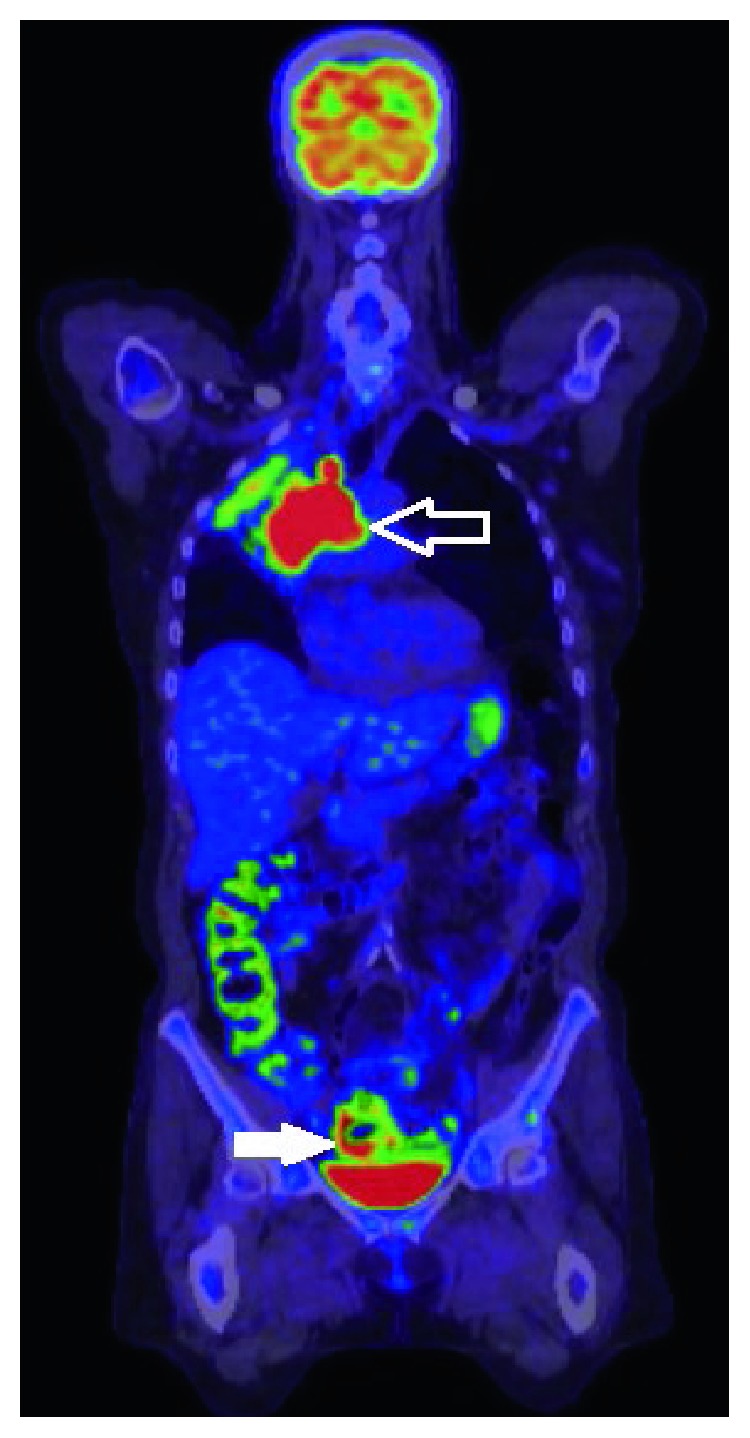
FDG-PET scan with an extensive right upper lobar and mediastinal mass in keeping with primary non-small-cell lung cancer (arrow). Intense heterogenous uptake in the sigmoid colon (white arrow), which could represent a synchronous malignancy or complication secondary to diverticular disease.
